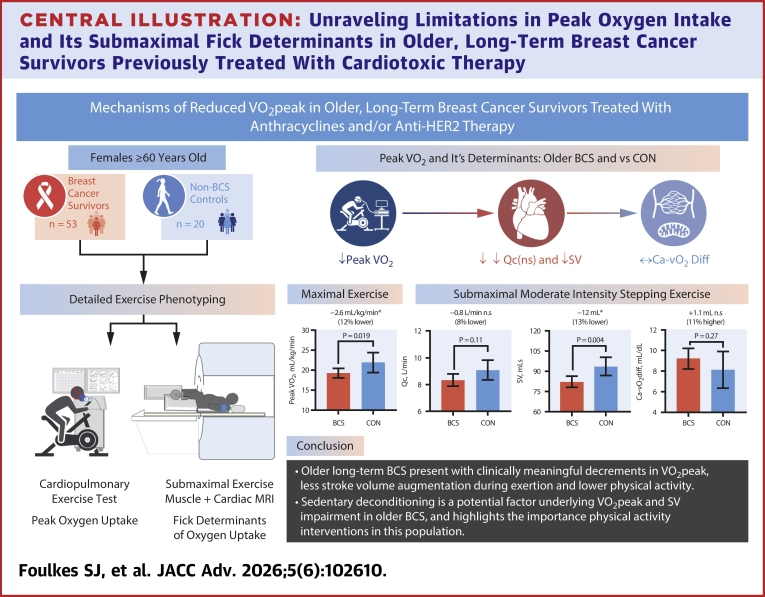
Unraveling Limitations in Peak Oxygen Intake and Its Submaximal Fick Determinants in Older, Long-Term Breast Cancer Survivors Previously Treated With Cardiotoxic Therapy Created using BioRender. ∗*P* < 0.05 for BCS vs CON. BCS = breast cancer survivors; C(a-v)O_2_diff = arteriovenous oxygen content difference; CON = controls; HER2 = human epidermal growth factor receptor 2; MRI = magnetic resonance imaging; n.s = not significant for BCS vs CON; Qc = cardiac output; SV = stroke volume; VO_2_ = oxygen intake; VO_2_peak = peak oxygen intake.
